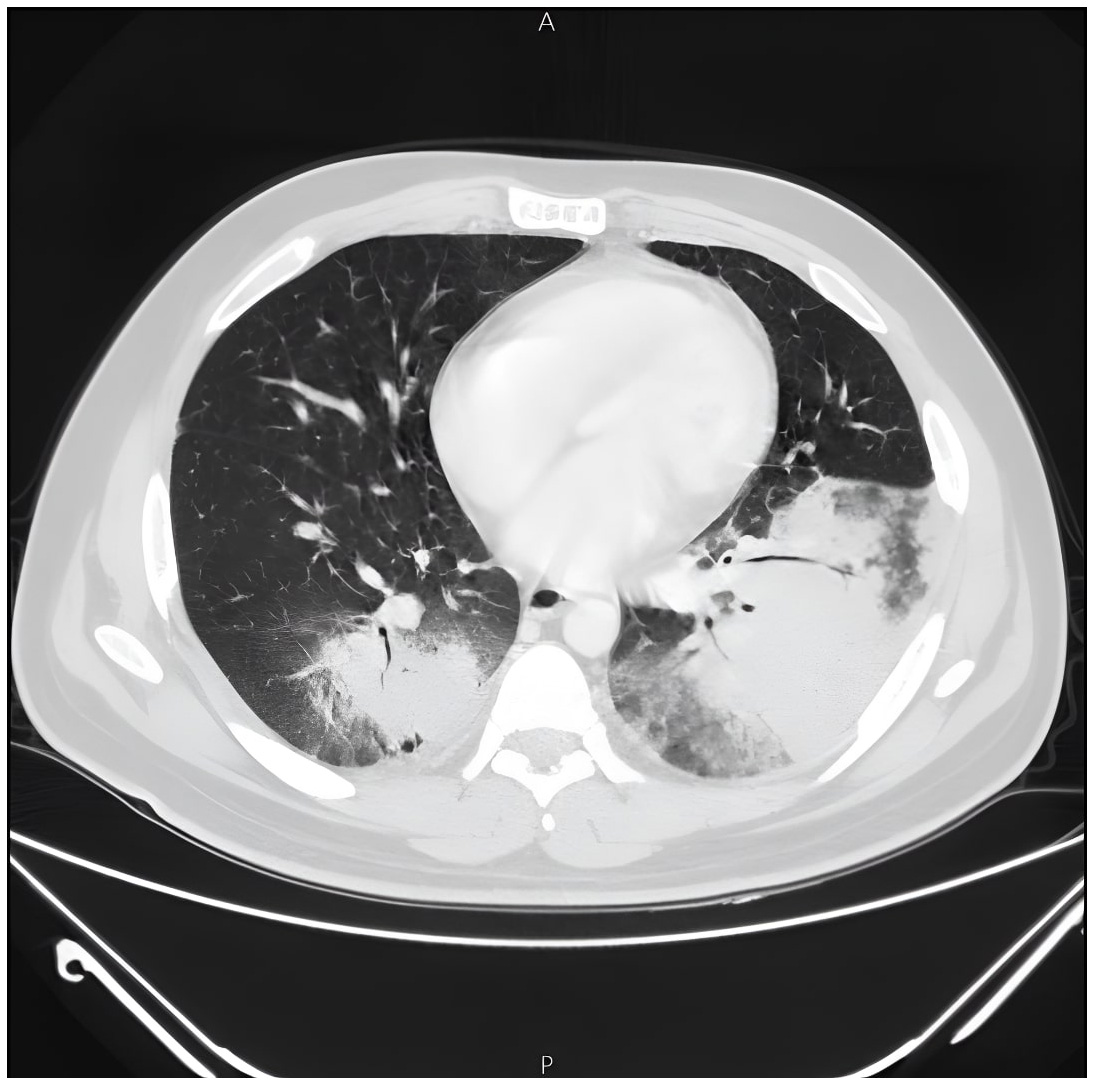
High resolution computed tomography scan of the chest showing patchy consolidations with a predominantly subpleural and/or peribronchial distribution likely suggesting Cryptogenic Organizing Pneumonia.
